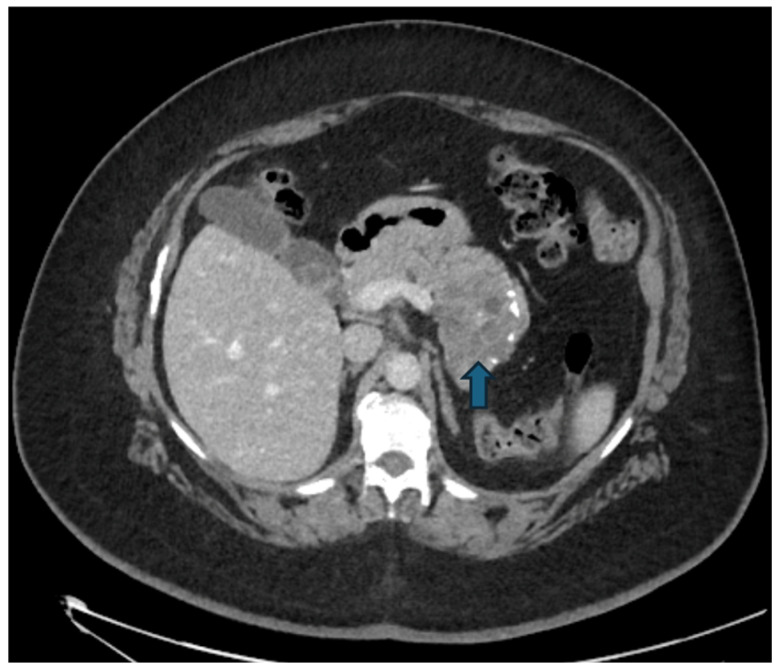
CT scan (portal venous phase) of a 67-year-old woman. A hypodense lesion is observed in the pancreatic body (arrow), with numerous thin, partially calcified internal septa, producing a characteristic “honeycomb” appearance, consistent with a microcystic serous cystadenoma.
